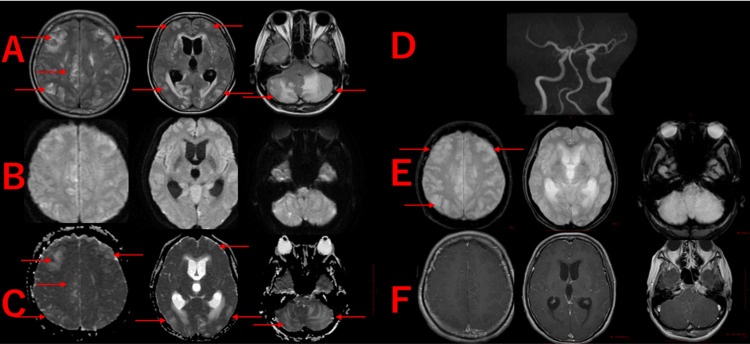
Brain Magnetic Resonance Imaging (MRI) on Initial Examination A: Fluid-attenuated inversion recovery (FLAIR) - High-signal areas are observed mainly in the cerebellum and brainstem, extending to the cerebral cortex and white matter (red arrow), B: Diffusion-weighted imaging (DWI) - No high-signal areas are detected, C: Apparent diffusion coefficient (ADC) - High-signal areas are observed in the same regions as on FLAIR, indicating vasogenic edema (red arrow), D: Magnetic resonance angiography (MRA) - No abnormalities are observed in the cerebral arteries, E: T2-weighted imaging - High-signal areas are noted (red arrow), F: Gadolinium-enhanced T1-weighted imaging (Gd-enhanced T1WI) - No contrast-enhancing tumors or thrombosis are observed.
